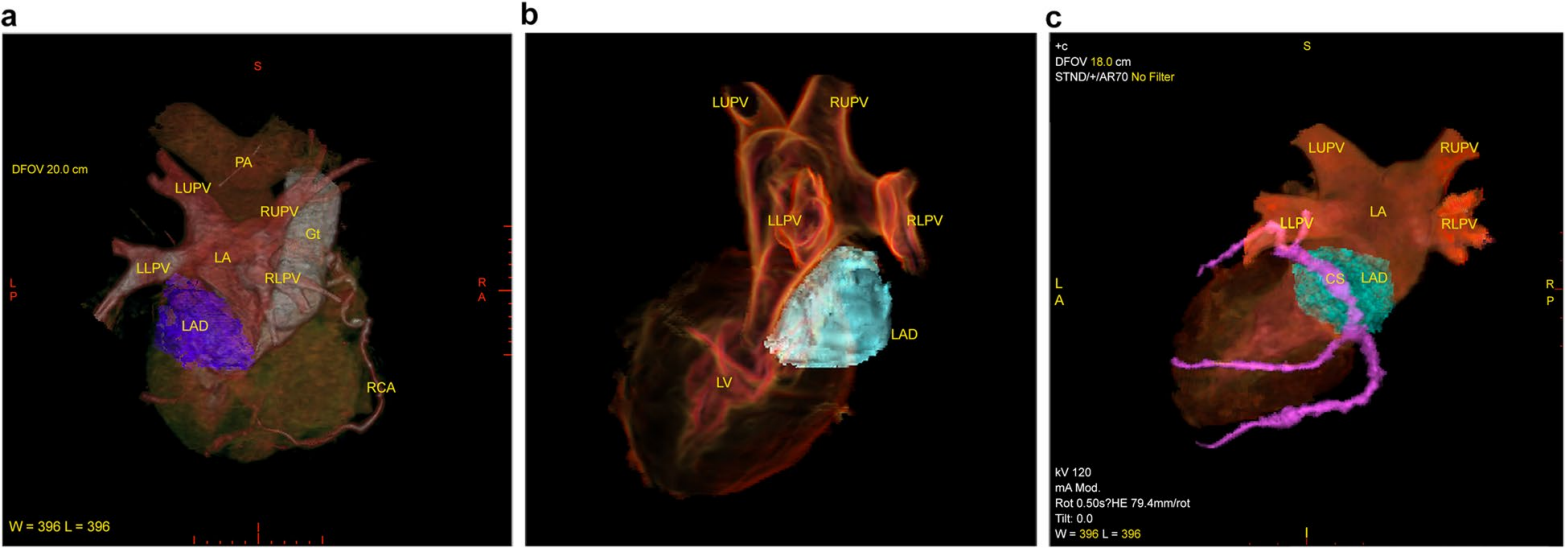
3D computed tomography (CT) identified the shape of the left atrial dissection (LAD) as a blue mass. a, b There is substantial space between the LAD and the pulmonary veins and c the LAD neighbors the coronary sinus. CS coronary sinus, Gt graft, LA left atrium, LAD left atrial dissection, LLPV left lower pulmonary vein, LUPV left upper pulmonary vein, LV left ventricle, PA pulmonary artery, RLPV right lower pulmonary vein, RUPV right upper pulmonary vein, RCA right coronary artery
